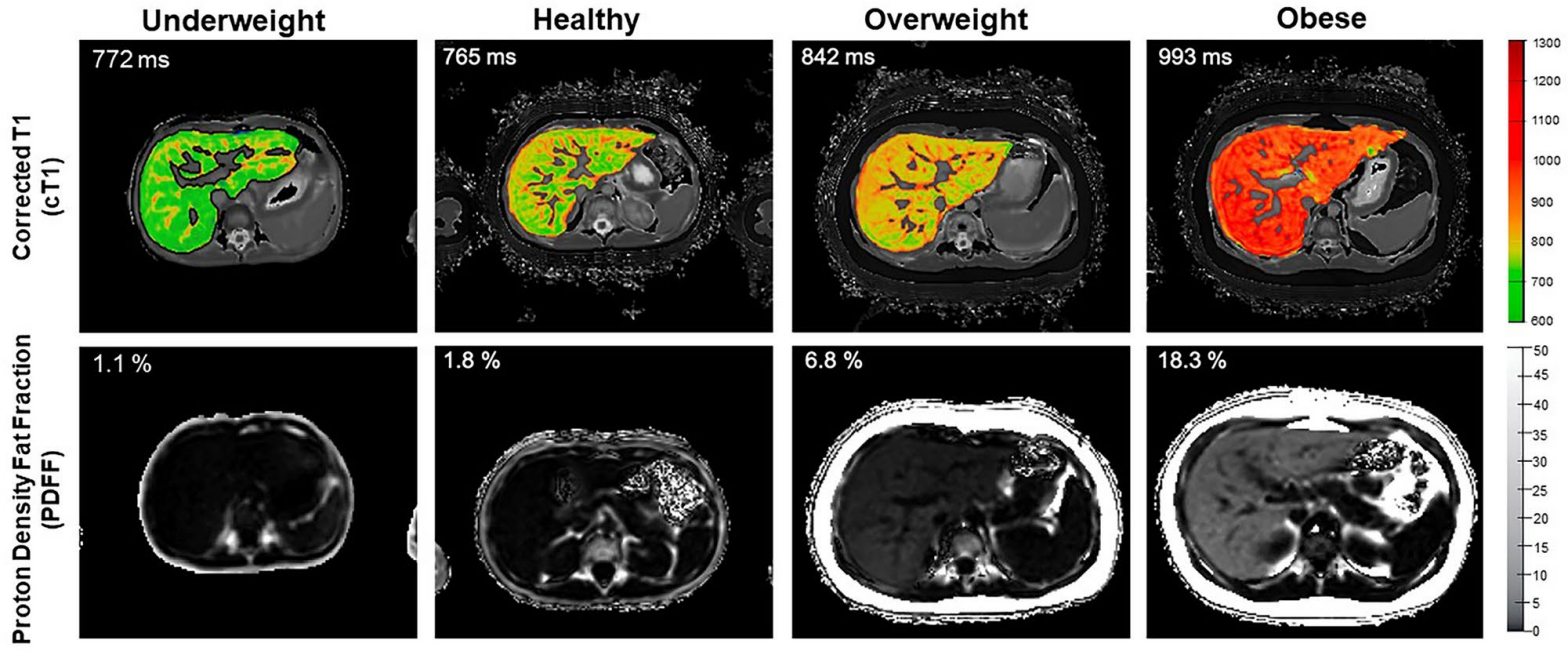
Typical transverse corrected T1 (cT1) and proton density fat fraction (PDFF) maps for underweight, healthy, overweight and obese participants calculated from multiparametric magnetic resonance imaging (mpMRI) data collected using LiverMultiScan (Perspectum Ltd, Oxford, UK) image acquisition protocol and MRI scanning sequences^44^.
